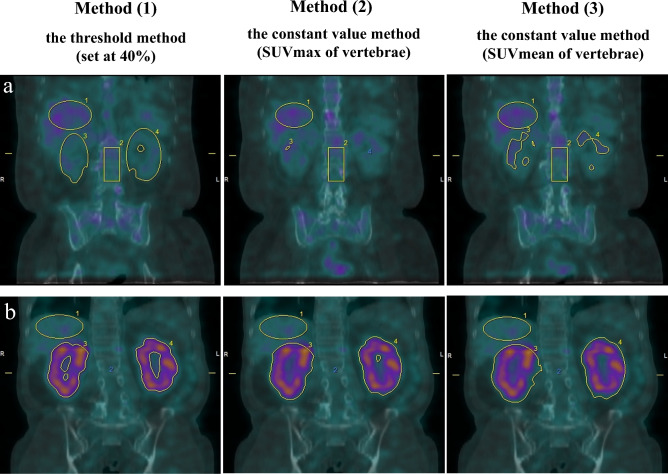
Three analysis methods for renal SUV_mean_ and ANV in grade 2 (a), grade 5 (b). (a) Grade 2: 40% = 0.92; vertebrae SUV_max_ = 2.3; vertebrae SUV_mean_ = 1.7. (b) Grade 5: 40% = 0.64; vertebrae SUV_max_ = 1.6; vertebrae SUV_mean_ = 1.2. In the case shown in Figure, the difference in VOI due to the difference in threshold settings is not a big difference in grade 5, but there is a big difference in grade 2. Lower grades are more susceptible to threshold settings. Method (1): VOI is set wider than the actual renal uptake, and there is a possibility of overestimation. Method (2): The VOI is set narrower than the actual renal uptake, and there is a possibility of underestimation. Method (3): Among these three methods, renal uptake was the most appropriate to set as VOI.
